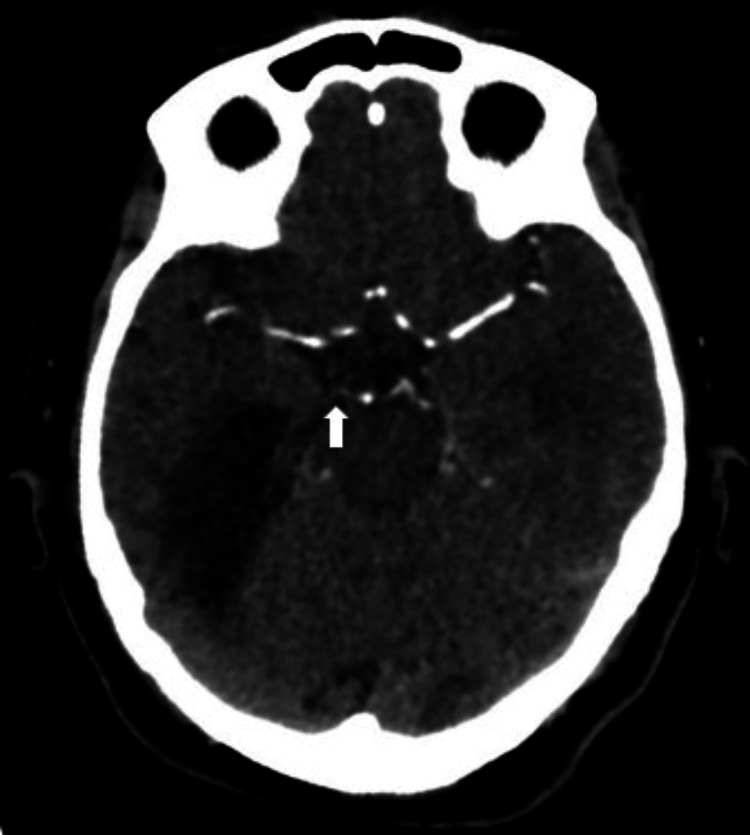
Cerebral CTA in the axial plane showed bilateral PCA stenoses (right > left) at the level of the circle of Willis. Small caliber and faint opacification were noted in the P1 segment of the right PCA (white arrow). CTA: computed tomography angiography; PCA: posterior cerebral artery.
